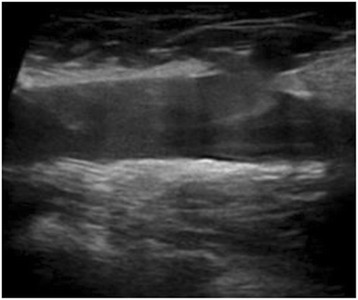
Ultrasound image of the complete resolution of the right common femoral vein thrombosis after 3-month therapy with rivaroxaban
